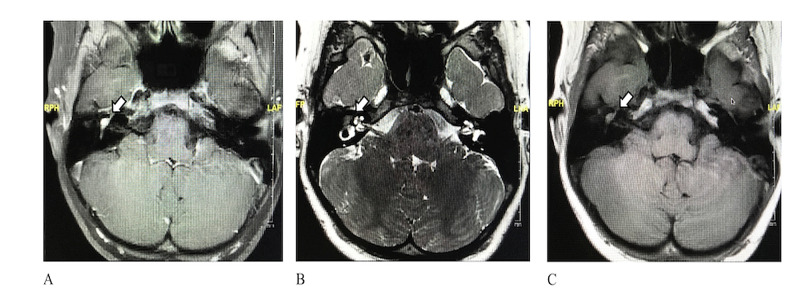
High-resolution MRI of the internal auditory canal in the axial plane. A. T1 post-contrast MRI shows enhancing lesion in the geniculate ganglion. B. T2 MRI shows isointense lesion in the geniculate ganglion. C. T1 pre-contrast MRI shows isointense lesion in the geniculate ganglion.
